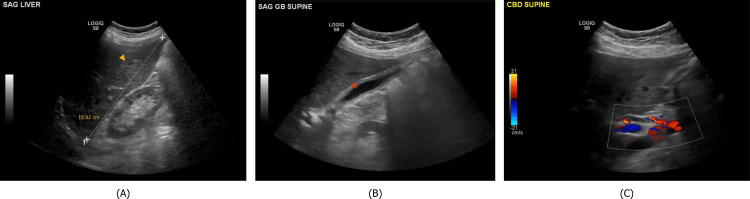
Liver ultrasonography obtained as part of investigation of the patient's elevated liver function tests. (A) Mild hepatomegaly is shown; the liver is measured at 18.92 cm. The yellow arrowhead points to a region of fatty metamorphosis. (B) Red arrowhead shows the gallbladder without any evidence of cholelithiasis. (C) Doppler imaging of the common bile duct reveals appropriate flow and no dilation.
